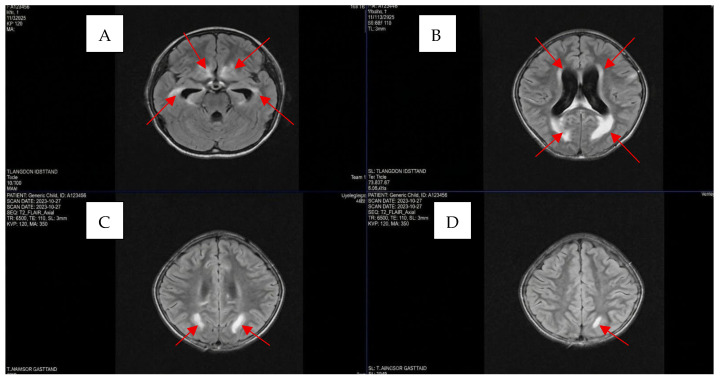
Non-contrast axial T2-FLAIR magnetic resonance imaging of the brain. Red arrows indicate the identified hyperintense lesions across the specified regions. (A) Bilateral heterogeneous hyperintensities located laterally to the ventral temporal horns, in the medial-dorsal floor of the frontal lobes, and at the junction of the optic chiasm. (B) Bilateral periventricular heterogeneous hyperintensities, along with posterior homogeneous hyperintensities displaying an arciform pattern in the parieto-occipital subcortical white matter (sparing the U-fibers). (C) Bilateral heterogeneous hyperintense lesions within the parietal subcortical white matter. (D) A solitary homogeneous hyperintense focus in the left parietal subcortical white matter, similarly sparing the U-fibers.
